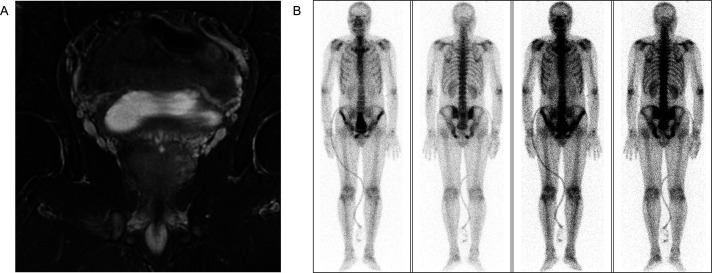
Preoperative imaging evaluation. (A) Prostate MRI. (B) Bone scintigraphy
